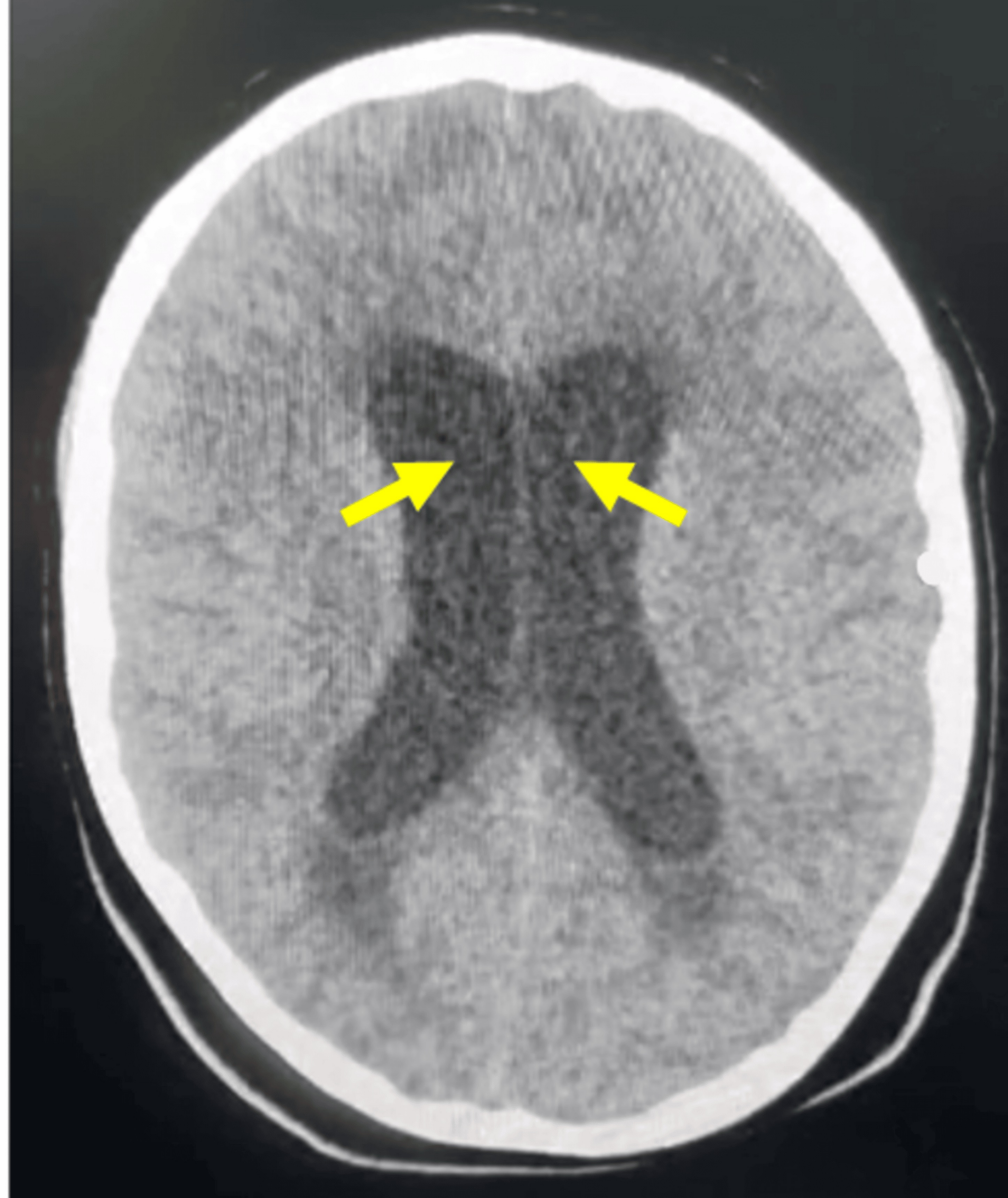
Axial head CT demonstrating obstructive hydrocephalus Axial computed tomography showing marked dilation of the lateral ventricles, particularly involving the frontal horns (arrows), consistent with obstructive hydrocephalus during the second hospitalization.
